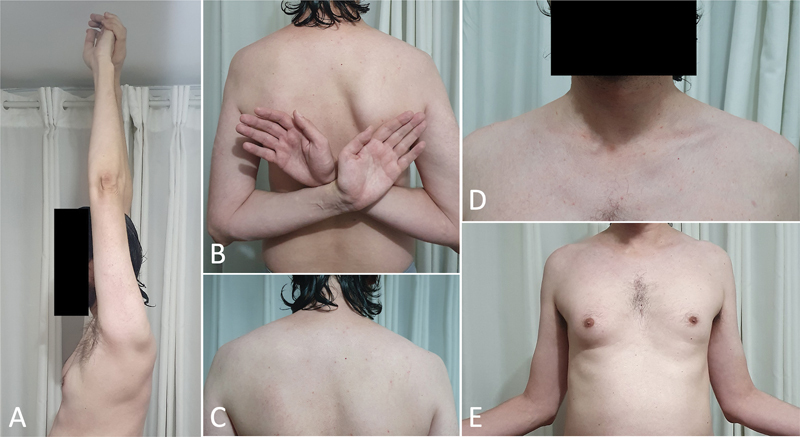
Radiographs of the left shoulder in anteroposterior (A), lateral (B), and axillary (C) views 18 years after the initial surgical treatment, demonstrating good appearance and no relevant changes. (A) Details of the glenoid contour and joint space. Source: authors' archive.
